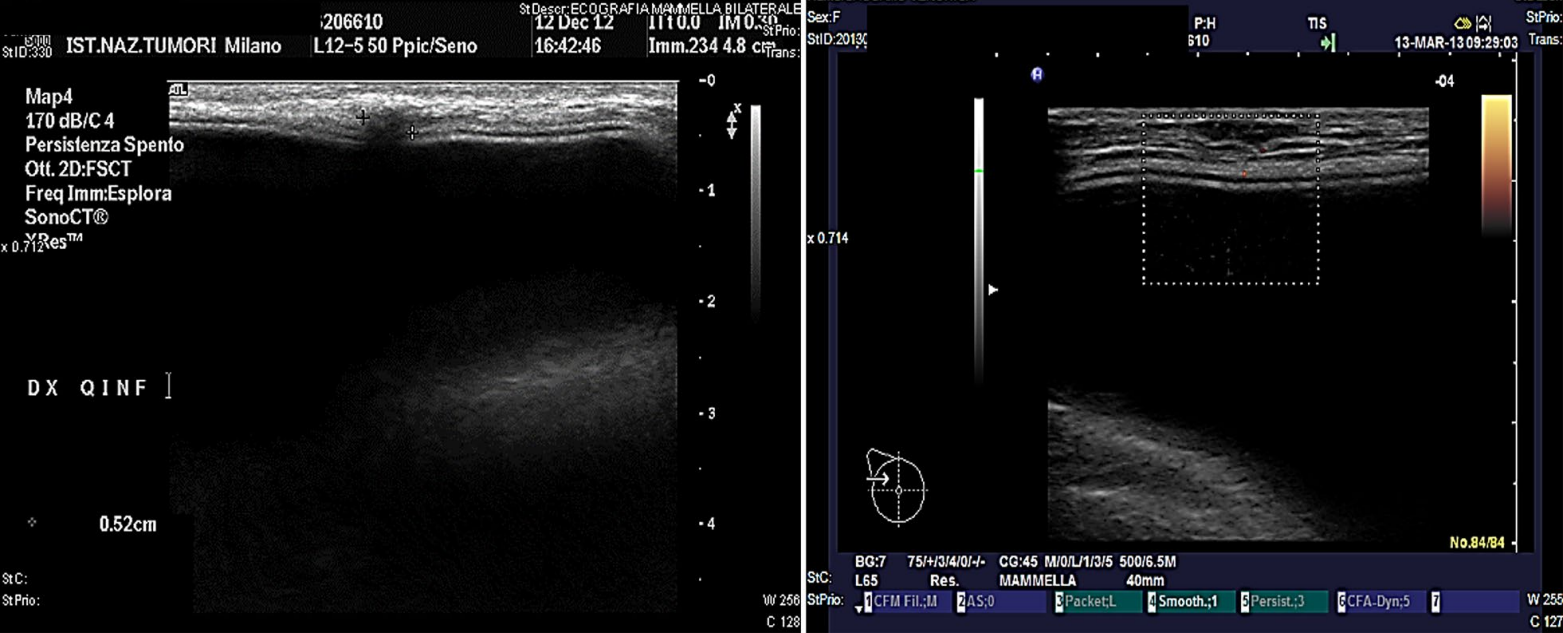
Ultrasound images. *Left* December 2012—the single nodule suggestive of granuloma in the lower-inner quadrant. *Right* March 2013—the nodule in the upper-outer quadrant, positive for recurrent DCIS
